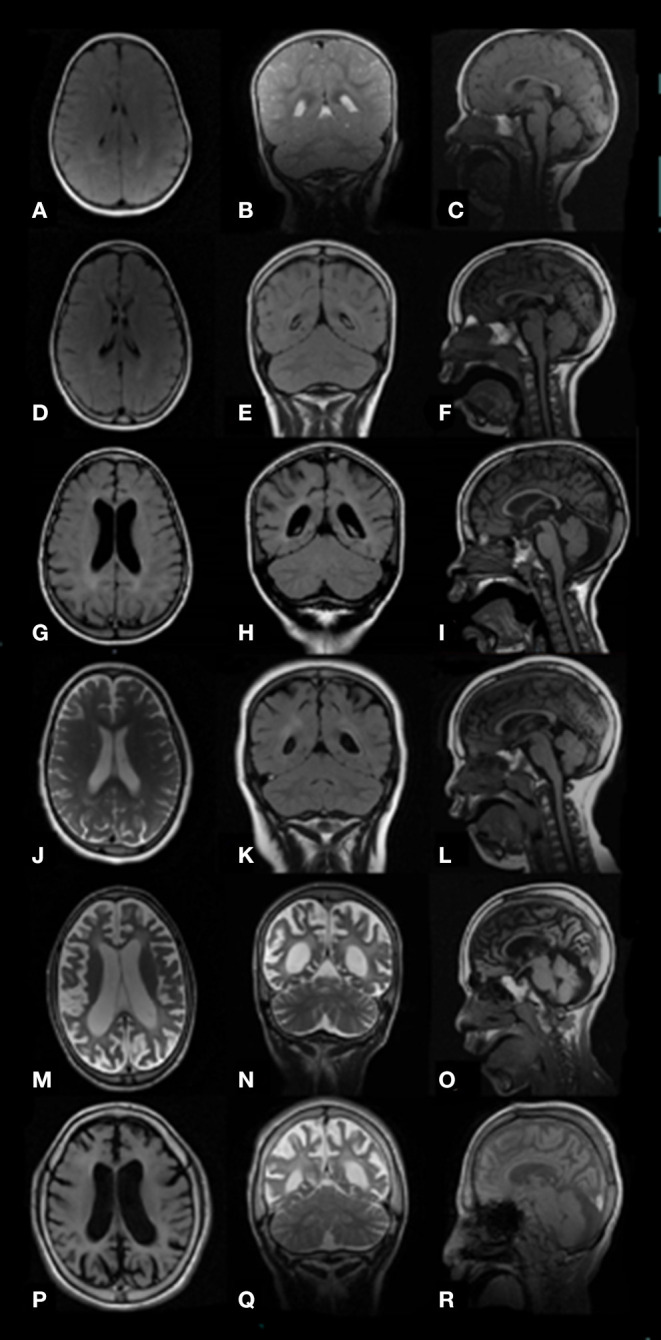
Brain images MRI (axial T2 or FLAIR, coronal T2 or FLAIR, and sagittal T1) of study patients with MPS III at different ages show progressive atrophic changes with enlargement of the lateral ventricles and progressive widening of the sulci secondary to brain volume loss. Pts. ID# 8 MPS III B, ages 19 months (A–C) and 6 years (D–F); ID# 3 MPS III A, age 7 years (G–I); ID# 9 MPS III B, age 8 years (J–L); ID# 3 MPS III A, age 11 years (M–O); ID# 10 MPS III B, age 12 years (P–R).
